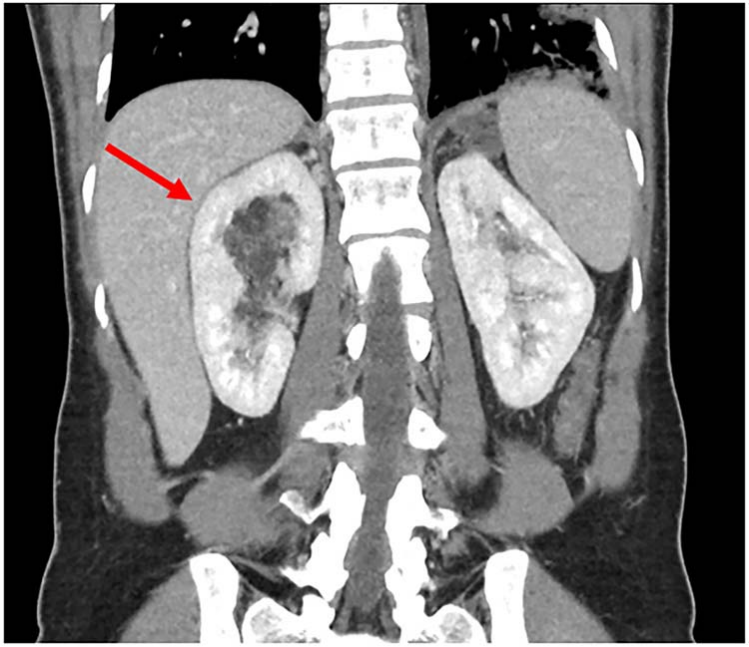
Preoperative CT showing renal AML.
